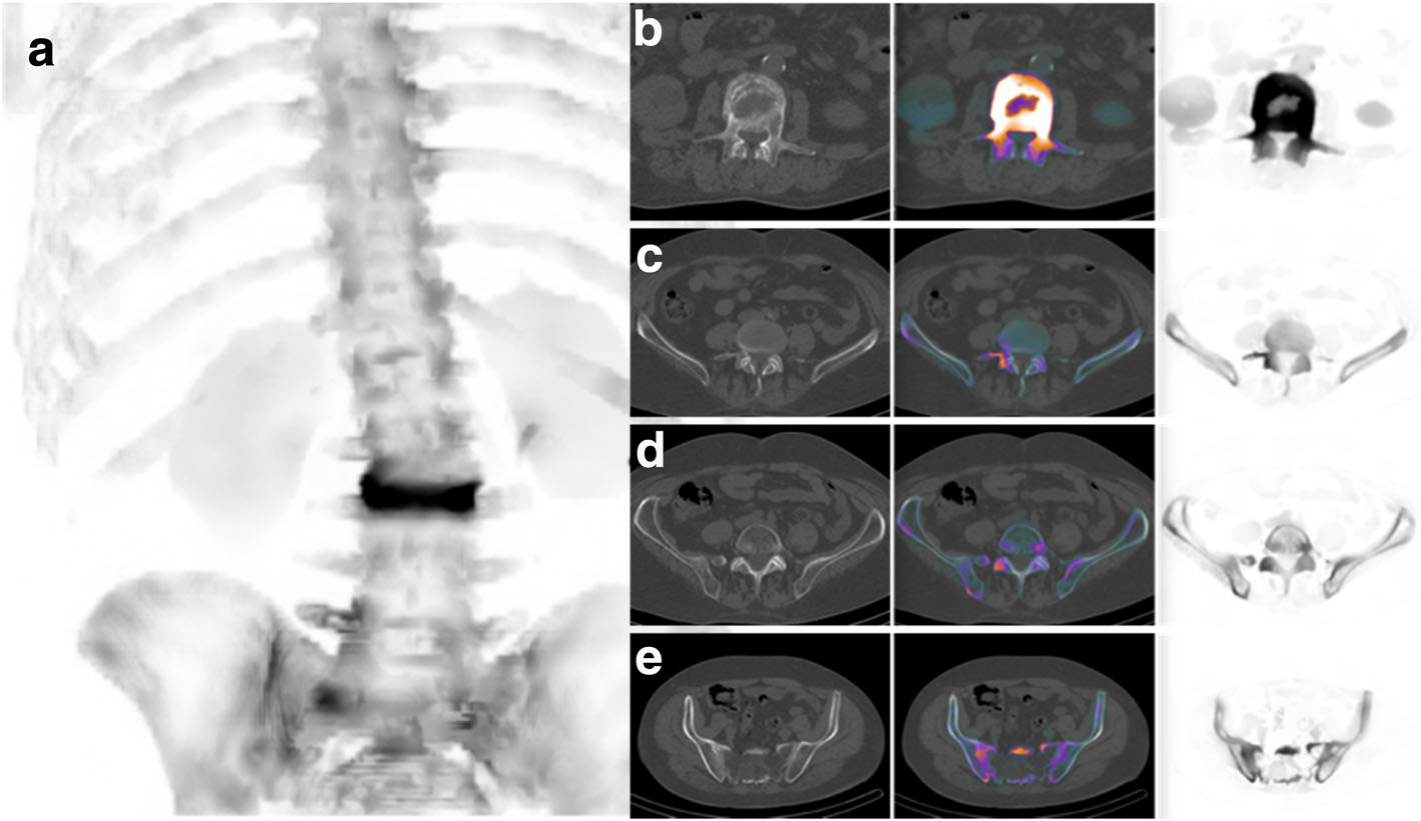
Example of an xSPECT Bone reconstruction providing SPECT images with bone anatomy appearance. The xSPECT Bone® maximum intensity projections (MIP) combines scintigraphic data with morphological data from the computed tomography. In this example, the scintigraphy was performed in order to explore an acute low back pain. The exclusive use of the xSPECT Bone® MIP (a) makes it possible to visualize on one image an uptake related to a fracture of a vertebral body responsible for spinal angulation, to identify the vertebra concerned (L3), to visualize an uptake localized on the fifth right transverse process extended to the zygapophysial joint, and to visualize an uptake of the last right zygapophysial joint and an uptake asymmetry of the sacroiliac joints. Transaxial image analysis confirms the fracture of the L3 vertebral body (b) and specifies its extension toward the pedicles. It also confirms the uptake of the fifth right transverse process (c), of the last right zygapophysial joint (d) and the uptake asymmetry of the sacroiliac joints (e)
